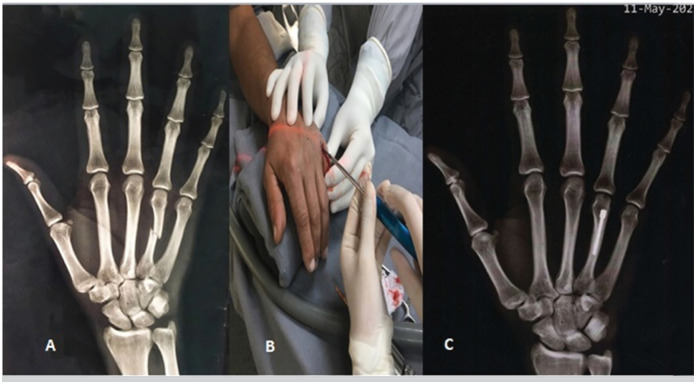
A) Anteroposterior radiograph – Diaphyseal fracture of the 4° metacarpal. B) IMCS surgical technique. C) Anteroposterior radiograph–Consolidation of the 4° metacarpal diaphyseal fracture.
